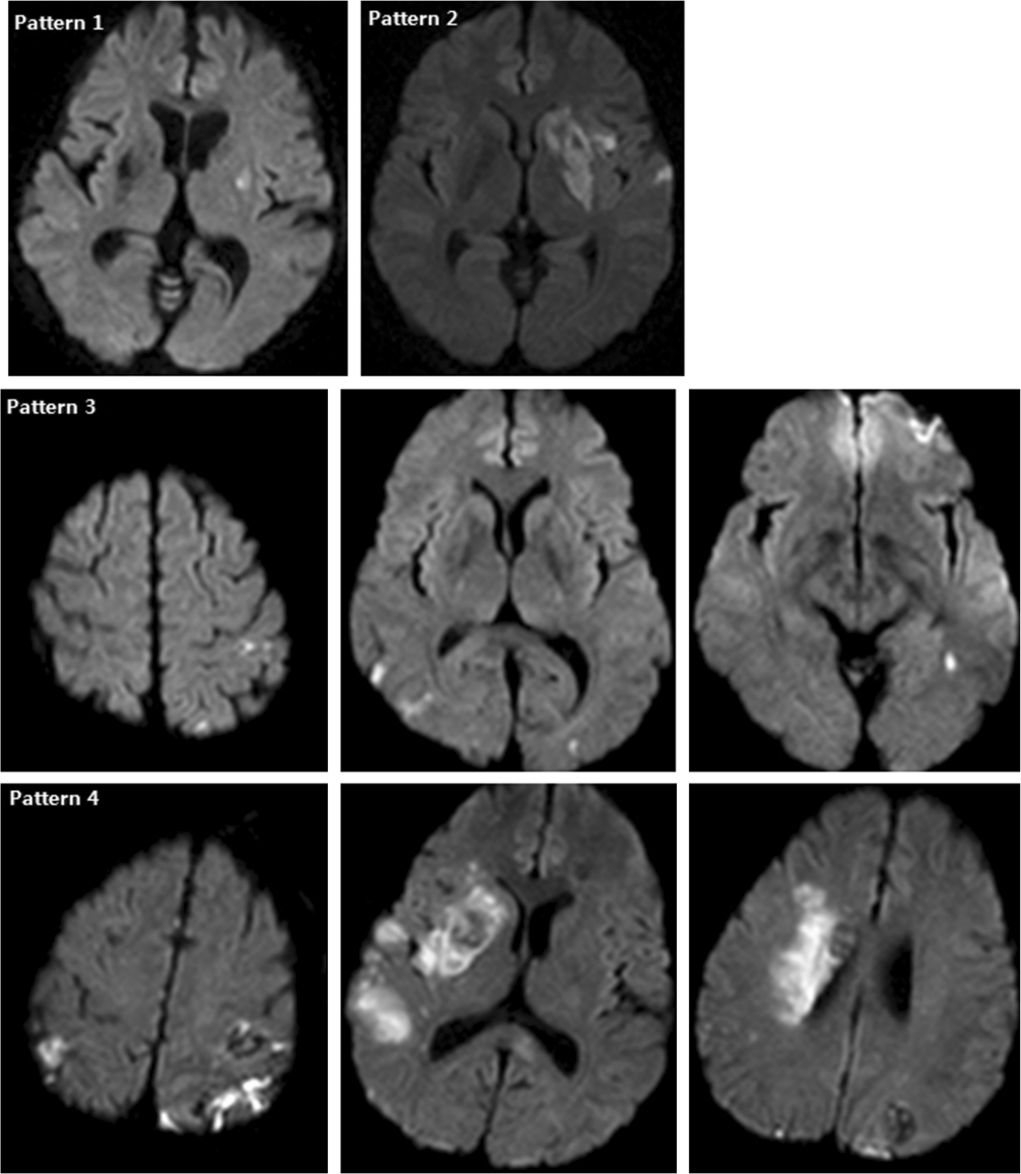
Patterns of acute embolic lesions on diffusion-weighted MRI in patients with infective endocarditis. Pattern 1 shown in a 63-year-old female with dysarthria and right hemiparesis, pattern 2 in a 43-year-old male with dysarthria and right hemiparesis, pattern 3 in a 59-year-old female with dysarthria alone, and pattern 4 in a 47-year-old male with confused mentality, dysarthria and left hemiplegia.
